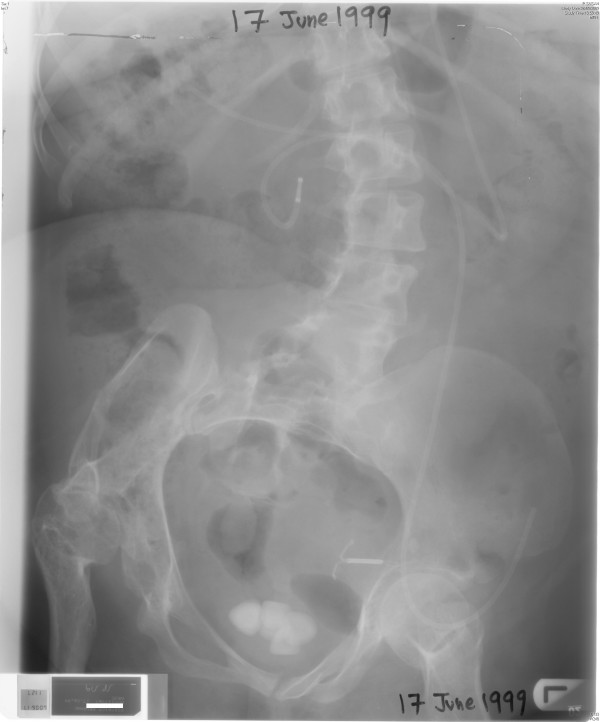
X-ray of abdomen, taken on 17 June 1999, showed several vesical calculi.
